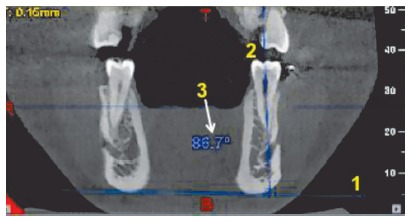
Calculation of buccolingual inclination of the teeth, on an 11×5 -cm CBCT section of the mandible.
